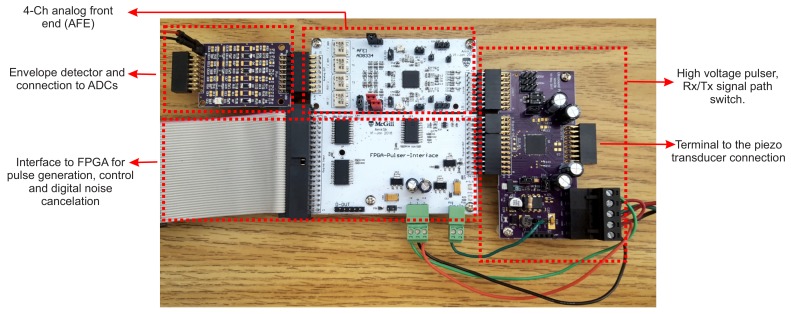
The designed 4-channel ultrasound system hardware setup consists of a high voltage pulser, an analogue front-end (AFE), an envelop detector and an ADC. It is interfaced with a Cyclone V FPGA board as a controller and data logger to the computer.
